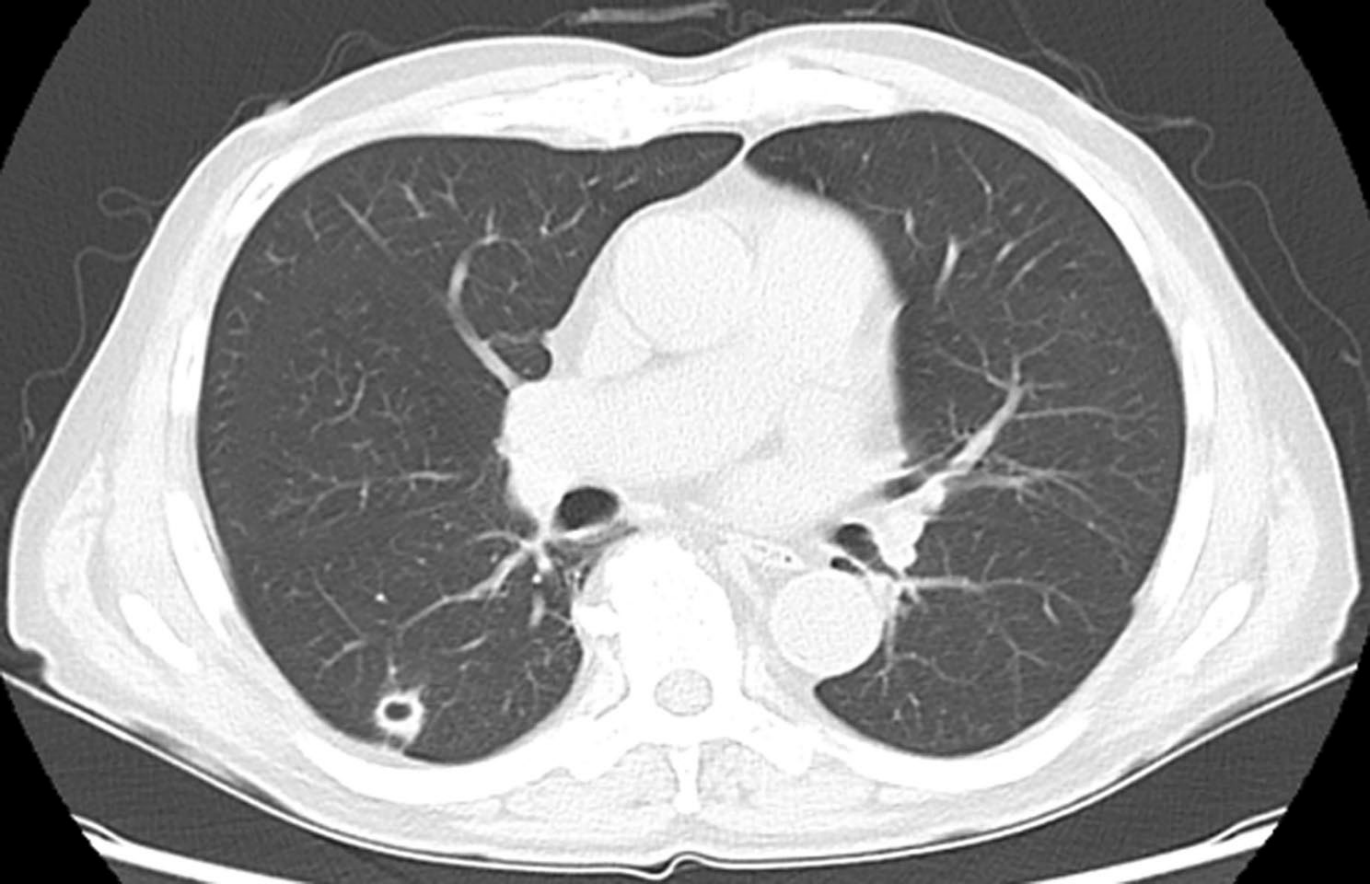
Chest computed tomography A 13-m nodule with a cavity is visible in S6 of the right lower lobe of the lung. The cavity wall was irregular and had a ground-glass opacity around it
